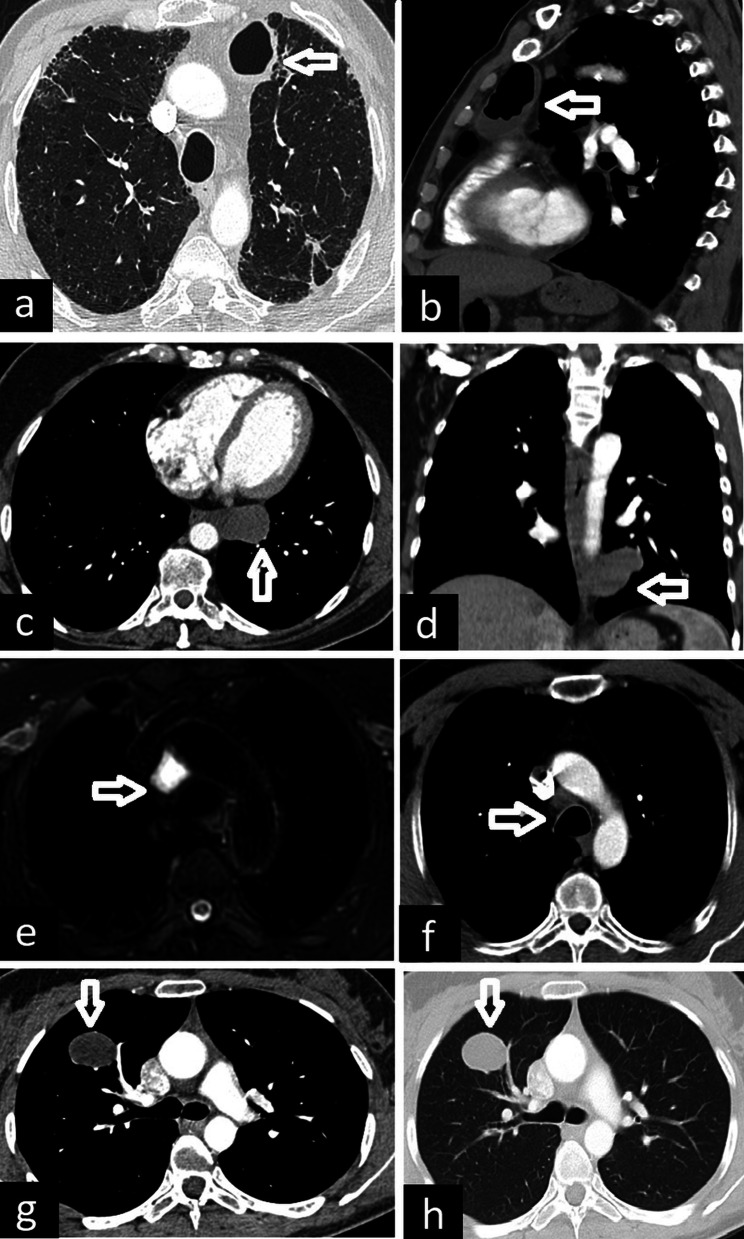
Differential diagnoses of bronchogenic cyst. Infected bullae on axial (a) and sagittal (b) CT images are observed. Esophageal duplication cyst on axial (c) and coronal (d) CT images, pericardial cyst on T2-weighted MRI (e) and axial CT image (f) and hydatid cyst on axial CT images (g, h) are considered in the differential diagnoses of bronchogenic cyst
